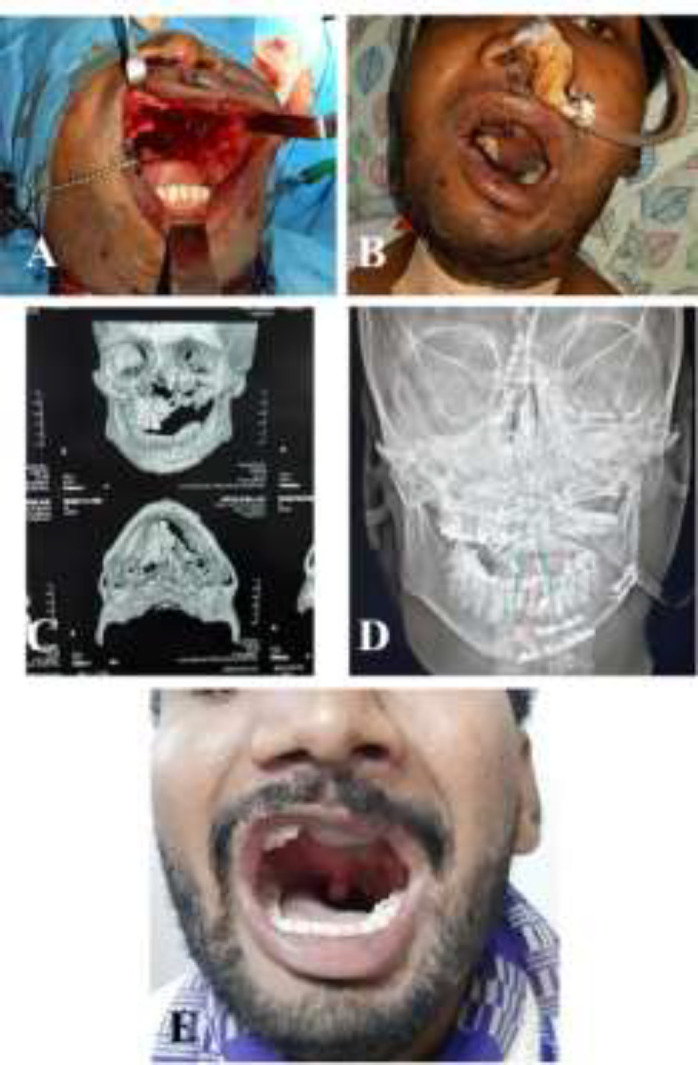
A: Post traumatic defect in the maxilla with loss of anterior wall in maxilla, alveolus, and more than half of the palate. B: Early post-operative picture of free fibula osteomusculocutaneous flap with flexor hallucis longus used to fill the antrum. C: Pre-operative CT scan image showing the defect. D: Post-operative X ray film showing the fibula. E: Late post-operative photo showing flap well settled
